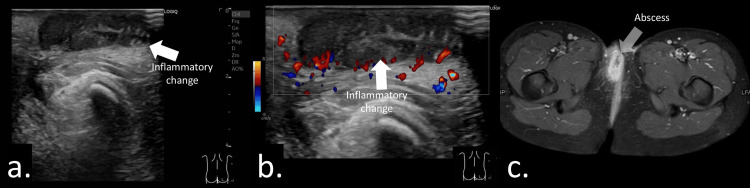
Inflammatory disease presenting with anal pain at the 11 o’clock position in a 12-year-old female. (a, b) Sagittal sonograms demonstrating a hypoechoic lesion surrounding a hypervascular area. No fluid collection was observed. (c) Axial contrast-enhanced MRI showing a small non-enhancing area surrounded by an enhancing region. The lesion measured 5 mm, and seton placement was not feasible due to its small size.
